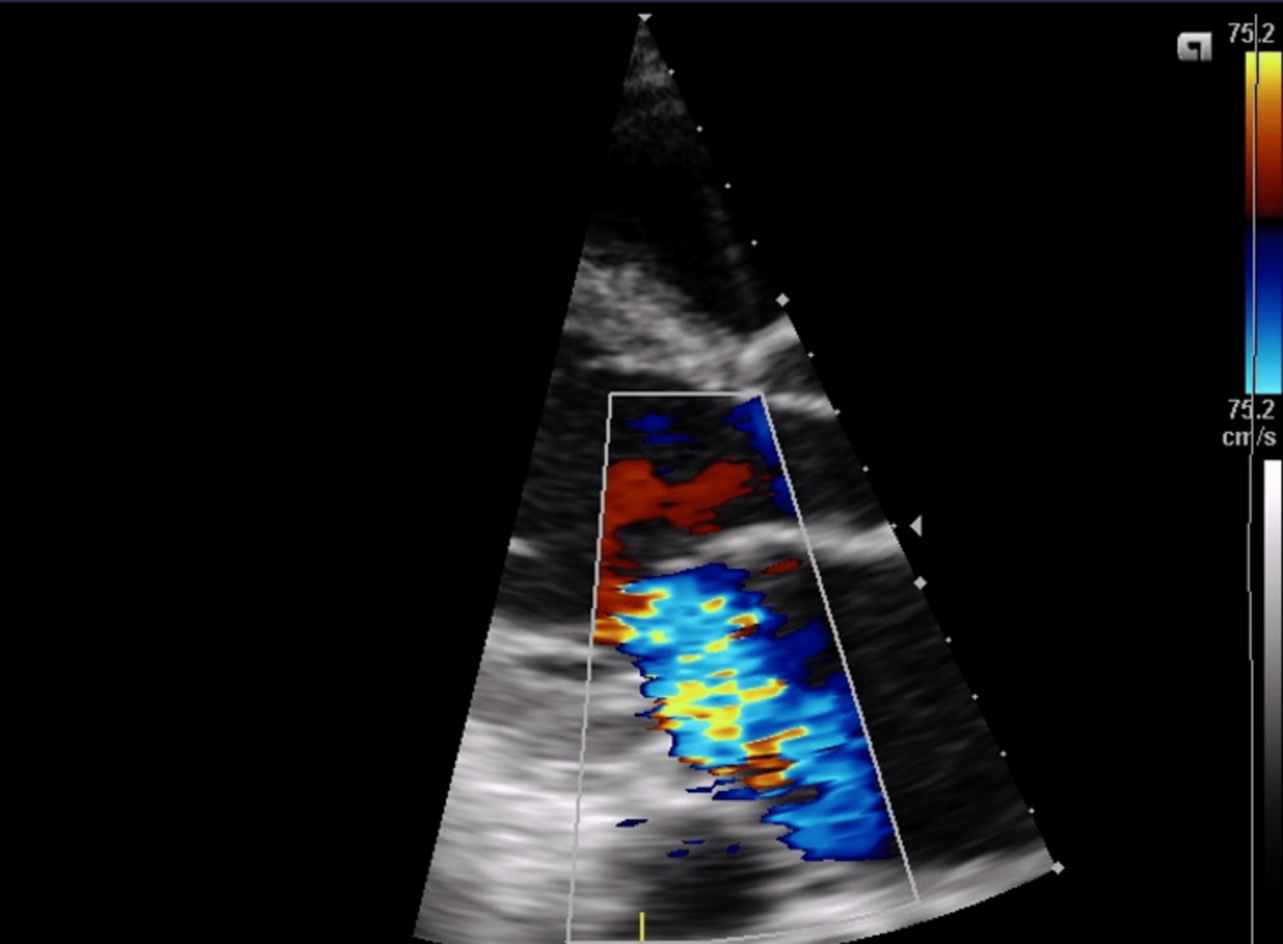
Parasternal long-axis image of the heart with color Doppler Imaging showing severe mitral regurgitation
